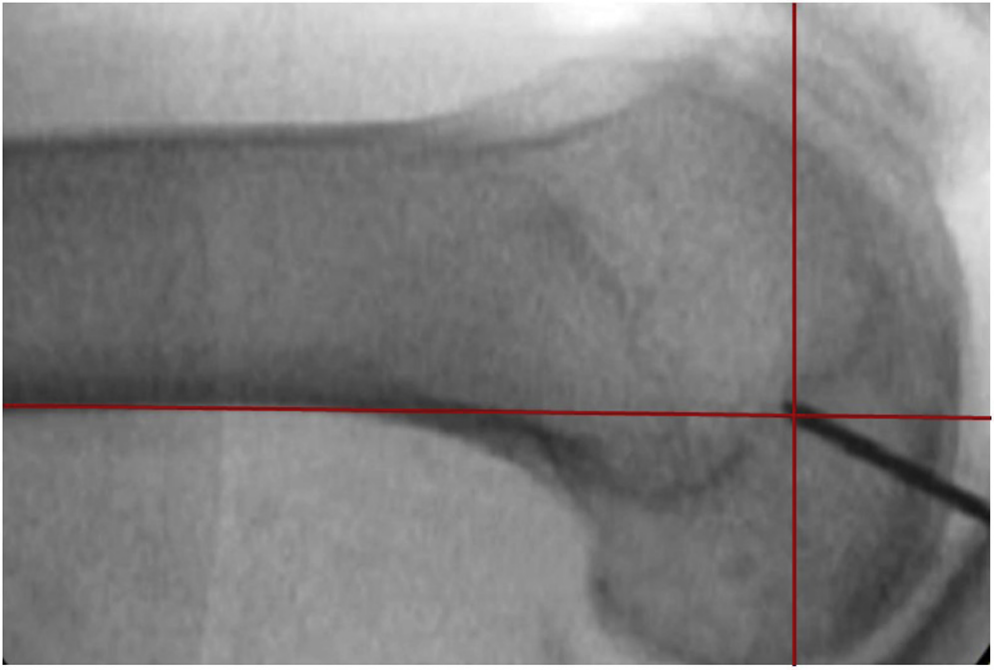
Radiographic landmarks for locating the femoral origin of the superficial medial collateral ligament (sMCL). A lateral view is shown of a right knee under fluoroscopy with a K-wire in the center of the femoral insertion of the sMCL. Line 1 is drawn parallel to the posterior aspect of the posterior femoral cortex (*y*-axis), and line 2 (*x*-axis) is drawn perpendicular to line 1, where line 1 intersects the Blumensaat line. The K-wire should be placed close to the intersection of the two lines in the proximal-anterior and proximal-posterior quadrant.^25^
